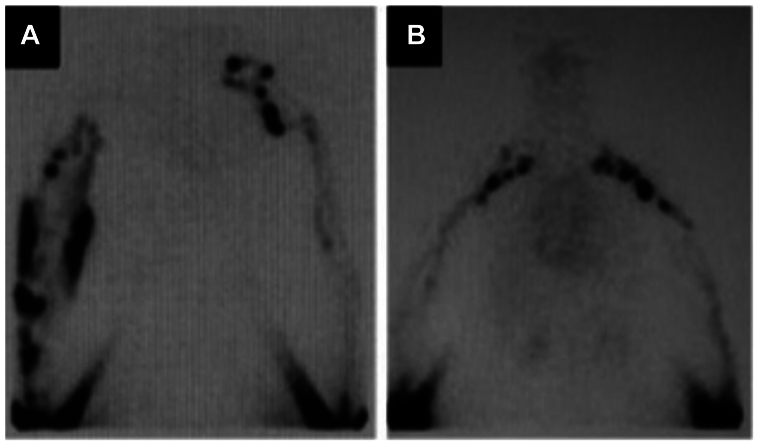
Sample lymphoscintigrams of the upper limbs of two breast cancer survivors with lymphedema (LED) of the right arms (A and B). However, the lymphoscintigram in panel B appears normal. Images reproduced from Mihara, et al^10^ under the Creative Commons Attribution license.
